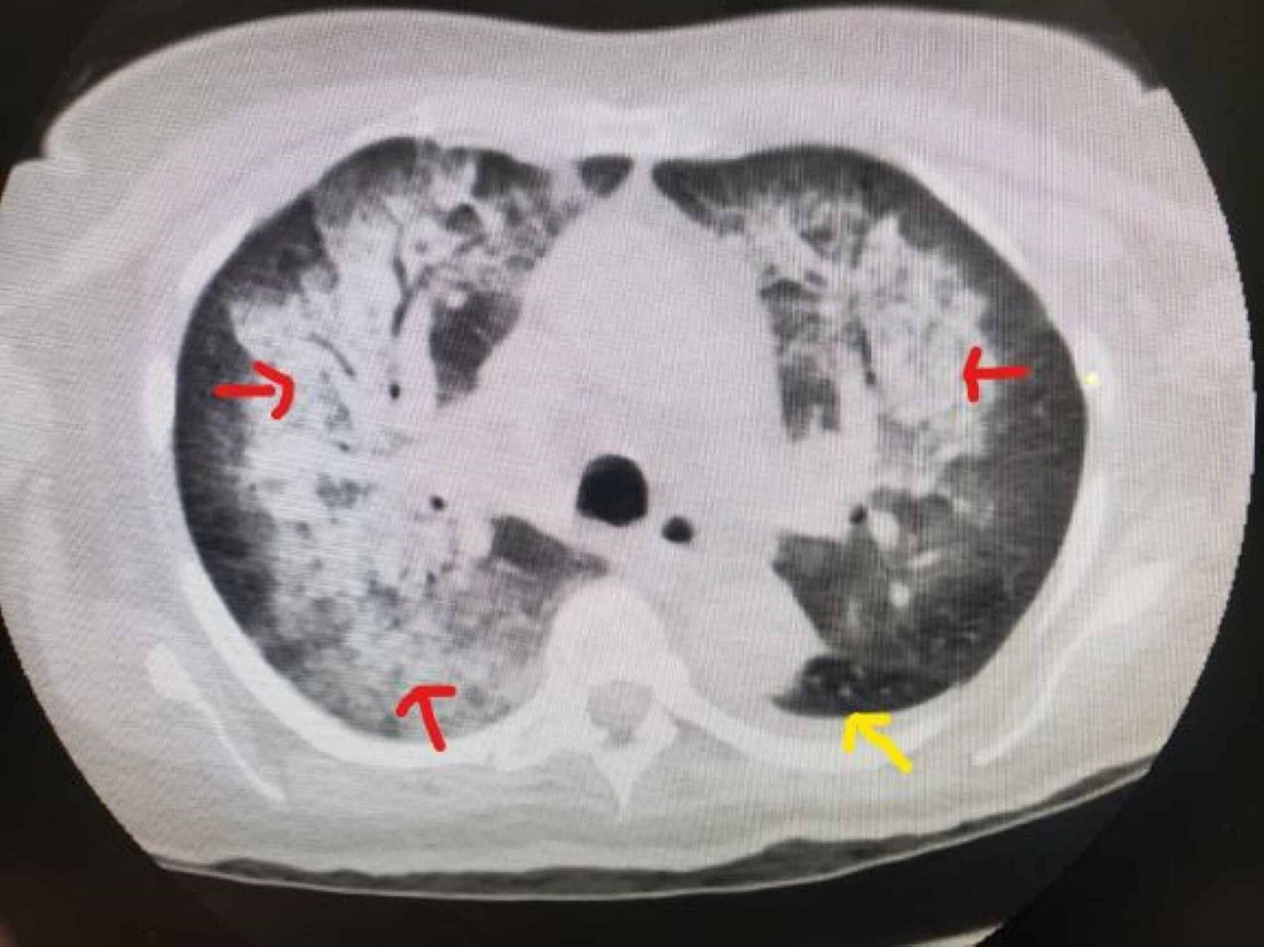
CT chest with diffuse mixed interstitial and alveolar airspace disease (red arrows) and small pleural effusion (yellow arrow)
